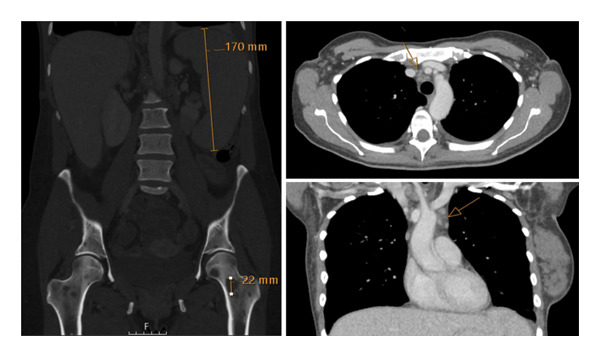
CT scan at the time of diagnosis, showing an enlarged spleen (17 cm), and multiple osteolytic lesions, the largest in the neck of the left femur (22 mm).
